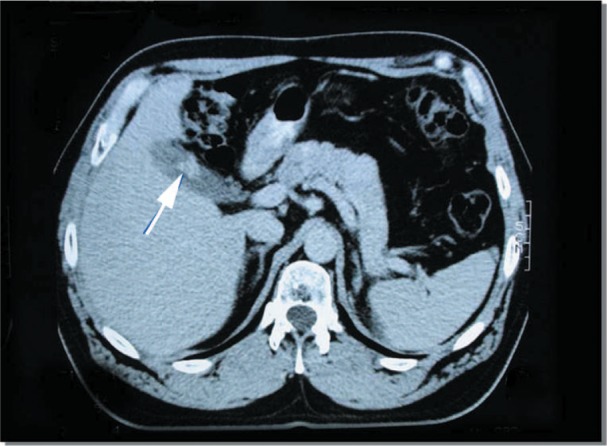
Case 1. Axial computed tomography image showing the polypoid structure adherent to the gallbladder wall (arrow).
